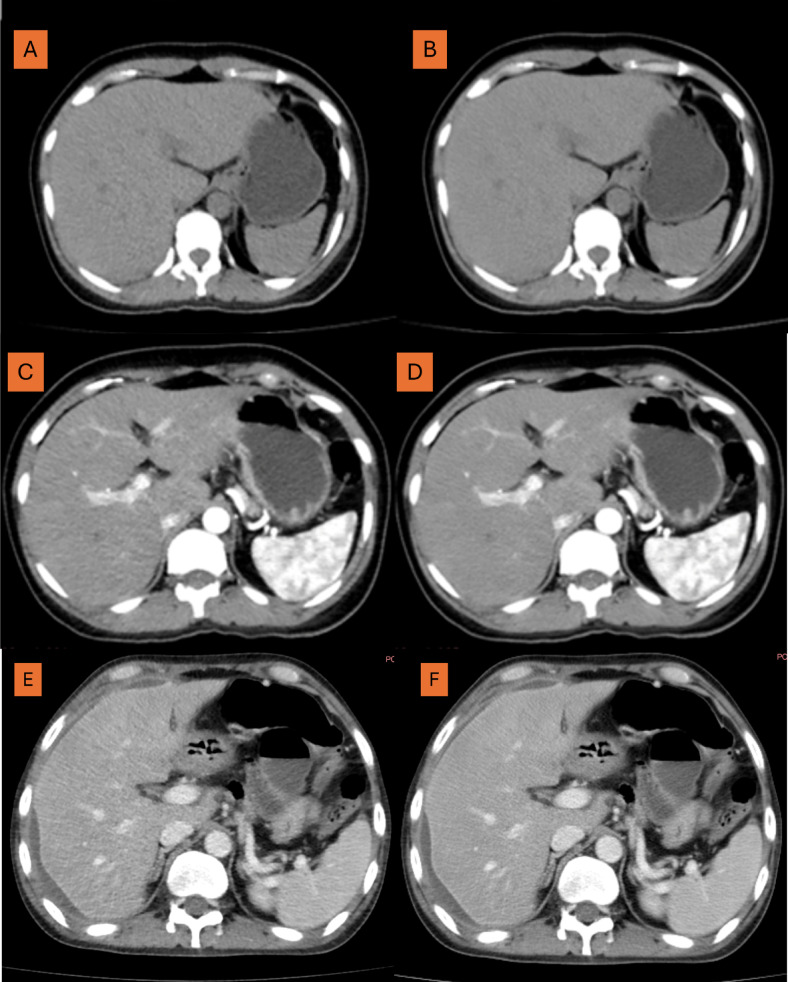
Non- contrast axial CT images of abdomen and pelvis in a 30- year-old male (BMI: 18.5 kg/m^2^). Image (A) reconstructed using iDose4 level 4 and image (B) reconstructed using PI (standard level). Images C and D representative of contrast arterial phase of 38 -year-old female (BMI: 17.3 kg/m^2^). Image (C) reconstructed using iDose4 level 4 and image (D) reconstructed using PI (standard level). Images E and F representative of contrast portal venous phase of 46 -year-old male (BMI: 17.7 kg/m^2^). Image (E) reconstructed using iDose4 level 4 and image (F) reconstructed using PI (standard level) Images are displayed with a window width of 350 and window level of 40.
